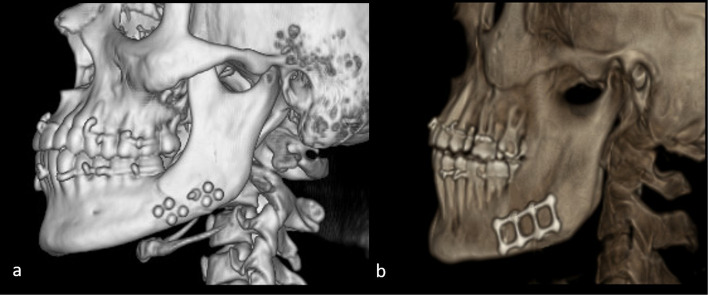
Three months postoperative 3D reconstruction view of CT-scan (a) 3D PEEK plate, b 3D Titanium plate
